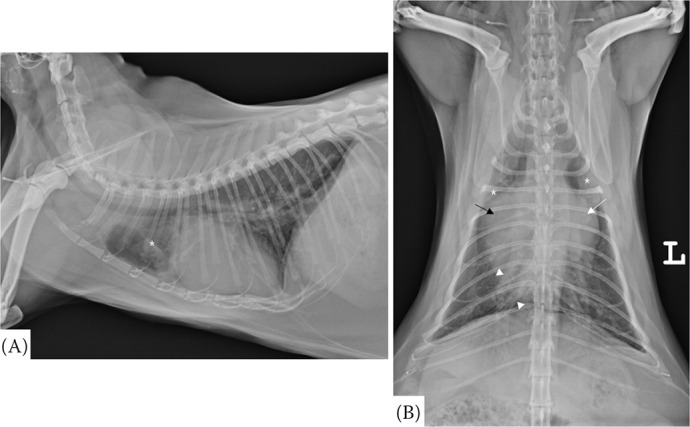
Right lateral (A) and ventrodorsal (B) thoracic radiographs showing severe cardiomegaly with enlargement of the left atrium (white arrow), right atrium (black arrow), right ventricle and dilation of the pulmonary arteries and veins (arrowheads) An alveolar pattern is observed in both the right and left cranial lung lobes (asterisks)
